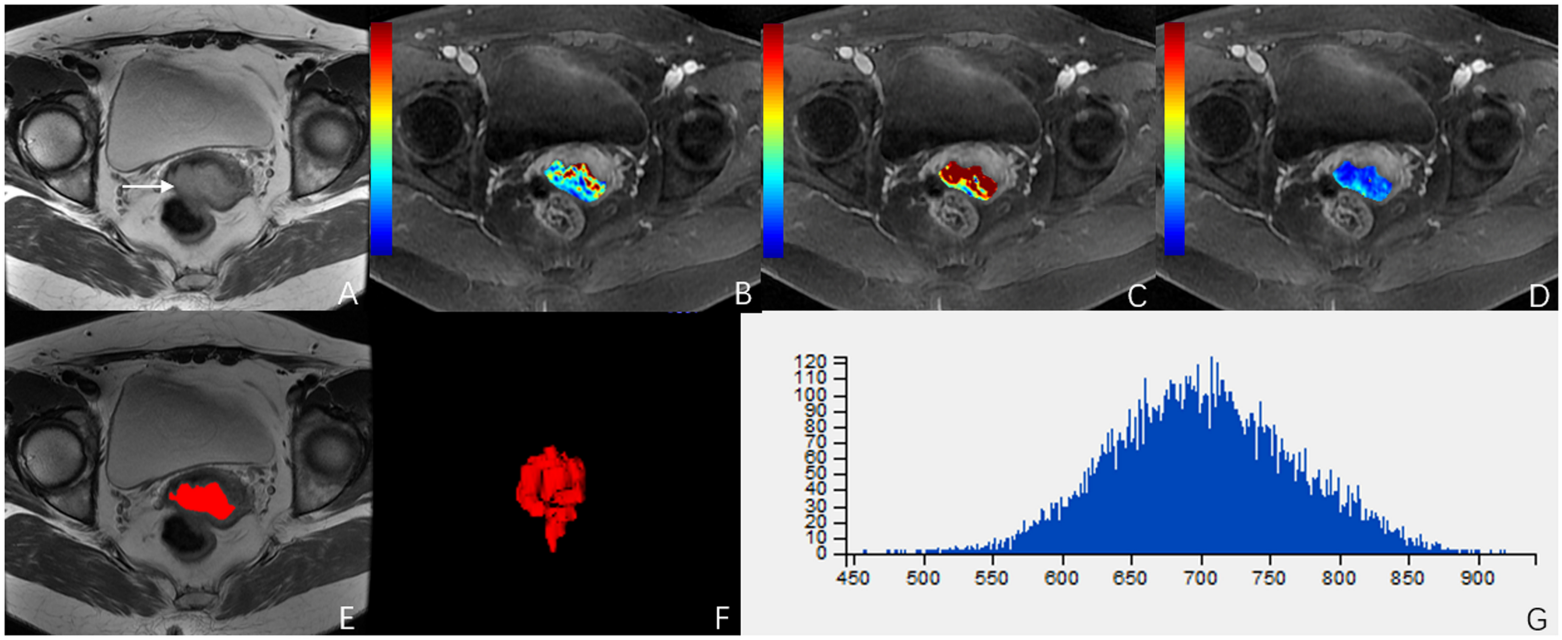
A 49-year-old woman with stage IIA cervical cancer. (A) T2-weighted image (T2WI) shows a slightly hyperintense cervical mass. (B–D) K^trans^, K_ep_, and V_e_ parametric maps are derived from DCE-MRI. The corresponding values are 0.196/min, 0.430/min, 0.396. (E,F) The volume of the tumor is drawn from T2WI. (G) Histogram map of the entire tumor.
